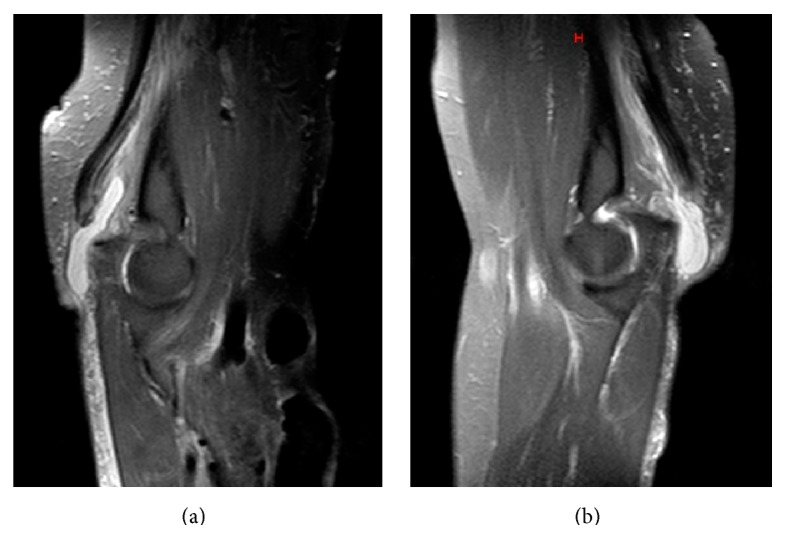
Magnetic resonance images of right (a) and left (b) elbows demonstrating complete avulsion of triceps tendon from the olecranon.
